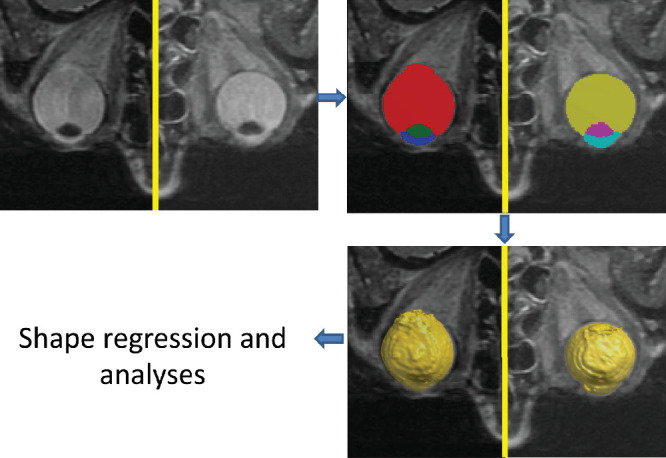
Example of segmentation, with a highly myopic eye on the left and an emmetropic eye on the right. The *left* figure shows the original acquired MRI image. The *upper right* figure depicts the separate areas that are segmented: the posterior segment in *red* and *yellow*, the lens in *green* and *purple* and the anterior chamber in *dark*
